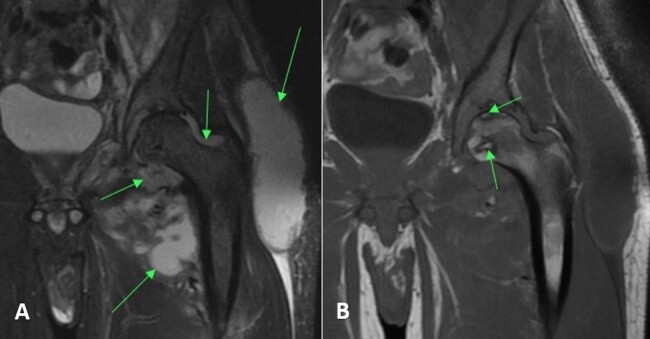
Case 1 hip MRI without contrast demonstrating (a) multiple abscesses and fluid collection (arrows) in the left hemipelvis with communicating septic arthritis of the hip on the T2-weighted (MRI sequence sensitive to tissue with high water content) sequence and (b) osteonecrosis of the femoral head (arrows) on the T1-weighted (MRI sequence sensitive to tissue high in lipid and protein) sequence.
